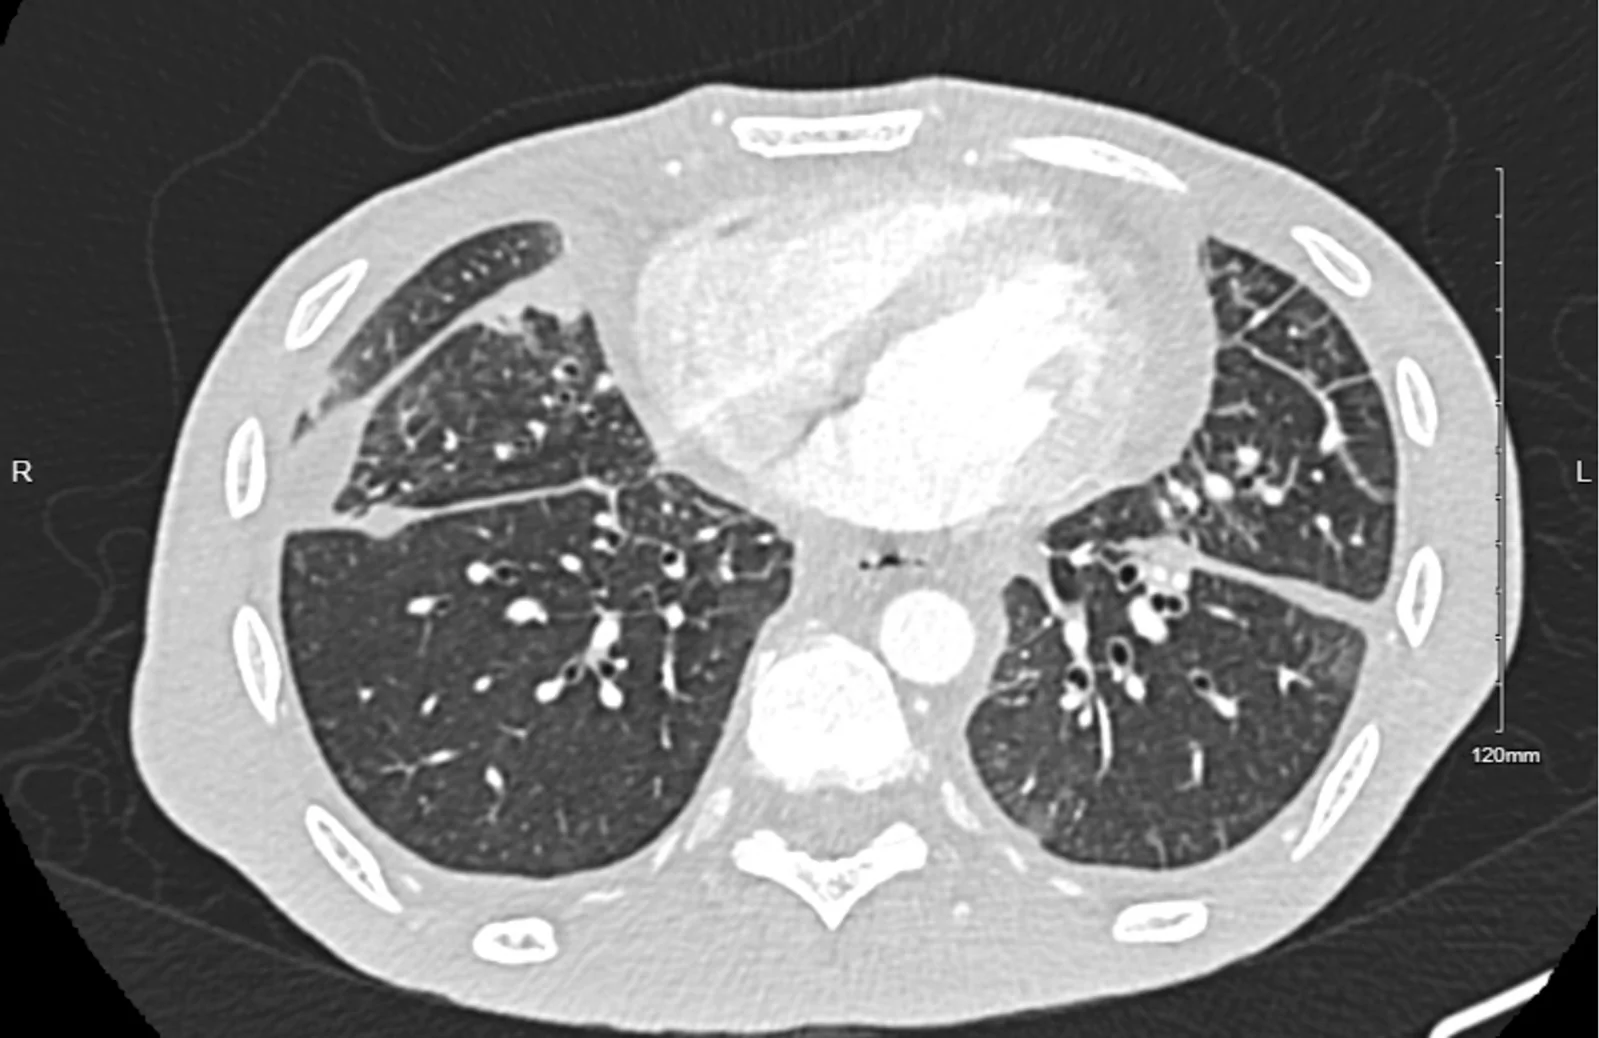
CT Angiogram of the Chest Demonstrating Right Middle Lobe Collapse and Bilateral Pleural Effusions Computed tomography angiography (CTA) of the chest obtained three days before hospital day 1, demonstrating collapse and consolidation of the medial segment of the right middle lobe with small bilateral pleural effusions.
